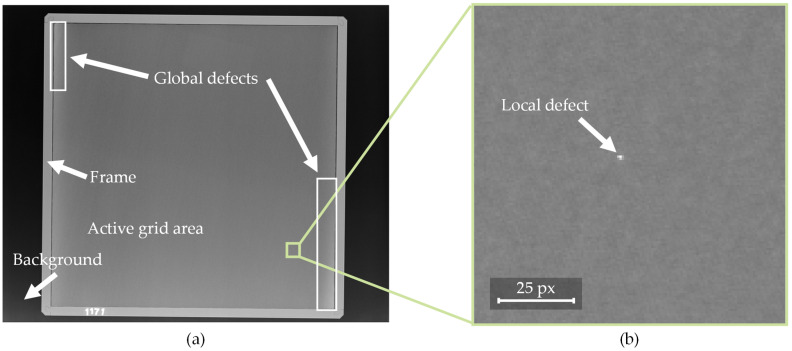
(a) Entire X-ray image including annotations for background, frame, active grid area, and two global defects; (b) subsection of (a) containing a highlighted local defect.
